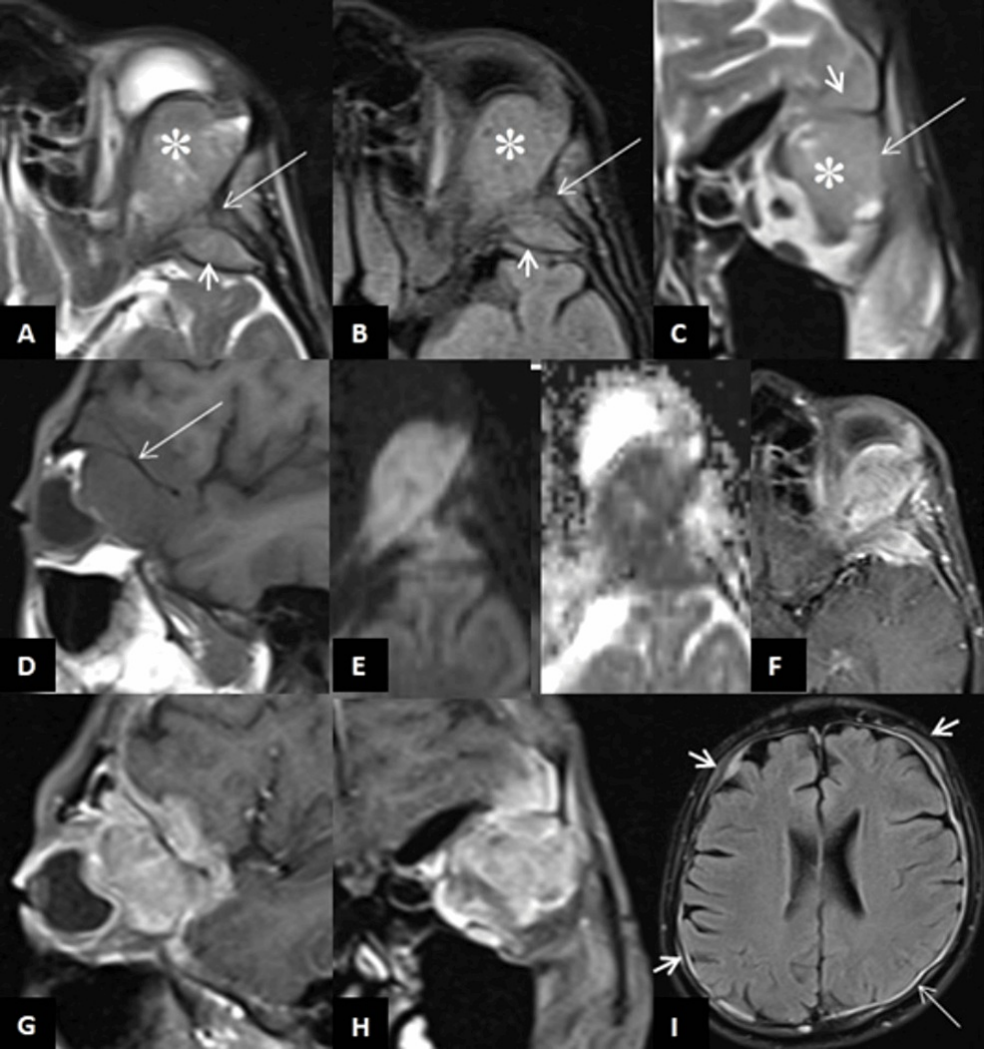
MRI images of the left orbital region (A-E) show a well-defined soft tissue lesion in the extra-conal compartment of the supero-lateral aspect of the left orbit. The lesion appears hyperintense on T2-weighted (A, C, asterisk) and FLAIR (B, asterisk) images, isointense to gray matter on T1-weighted images (D), and demonstrates diffusion restriction (E). There is an abnormal signal with erosions of the adjacent lateral wall of the left orbit, the lesser wing of the sphenoid, and the spheno-temporal buttress (A-D, long arrow), along with associated extra-dural soft tissue (A-C, short arrow). Heterogeneous enhancement of the orbital and extra-dural soft tissue lesions, as well as the involved bones, is noted on T1 fat-saturated images (F-H). Post-contrast fat-saturated axial FLAIR image (I) at the level of the body of the lateral ventricles also shows multiple abnormal calvarial lesions with adjacent soft tissue components (small arrows) and enhancing pachymeningeal thickening (long arrow). These findings are consistent with multiple skeletal metastases and a left extra-conal orbital soft tissue mass. FLAIR: fluid-attenuated inversion recovery
